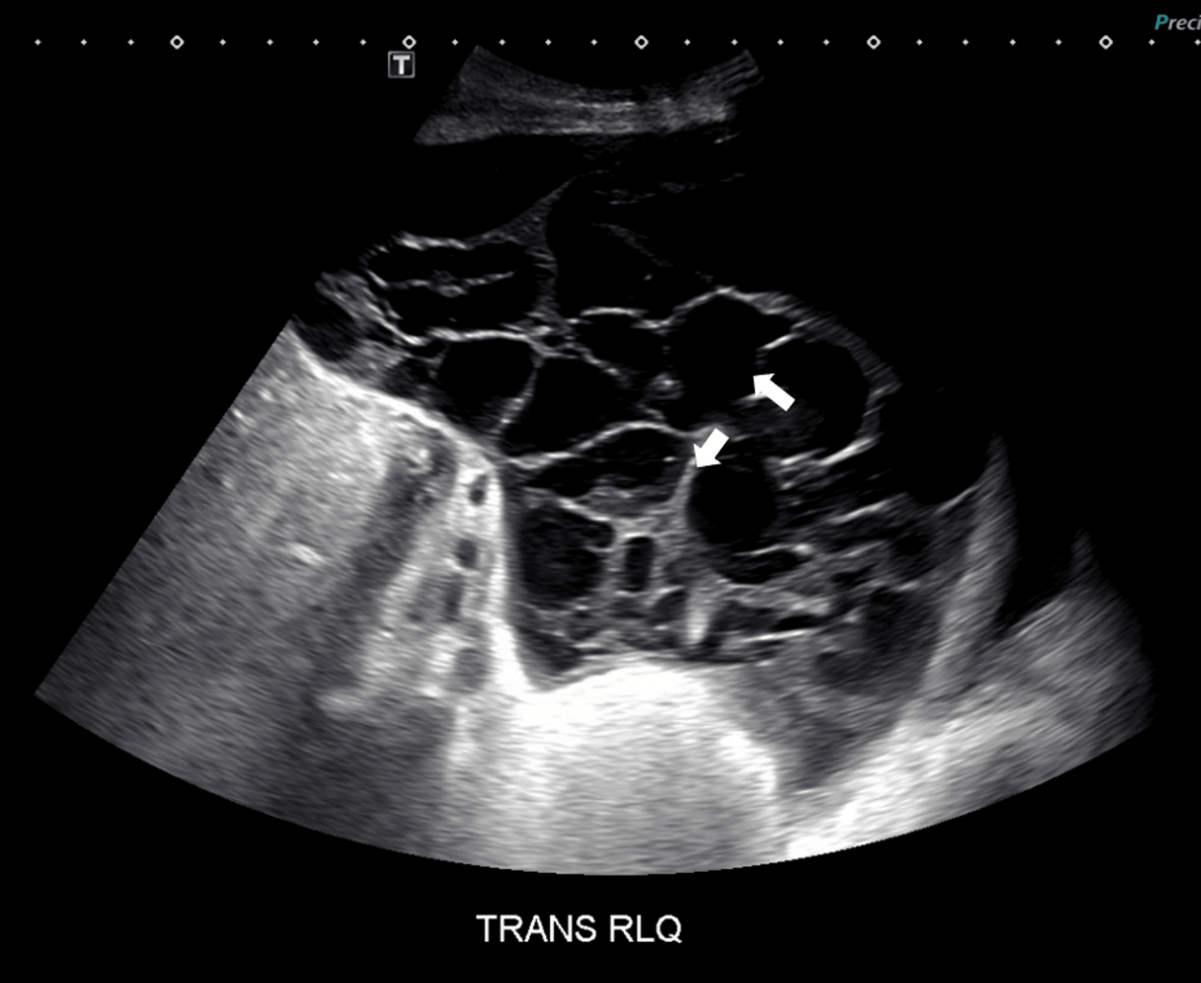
Abdominal ultrasound demonstrating loculated ascitic fluid before catheter-directed tPA administration. Arrows indicate septations between loculated ascitic fluid collections. tPA: Tissue Plasminogen Activator.
